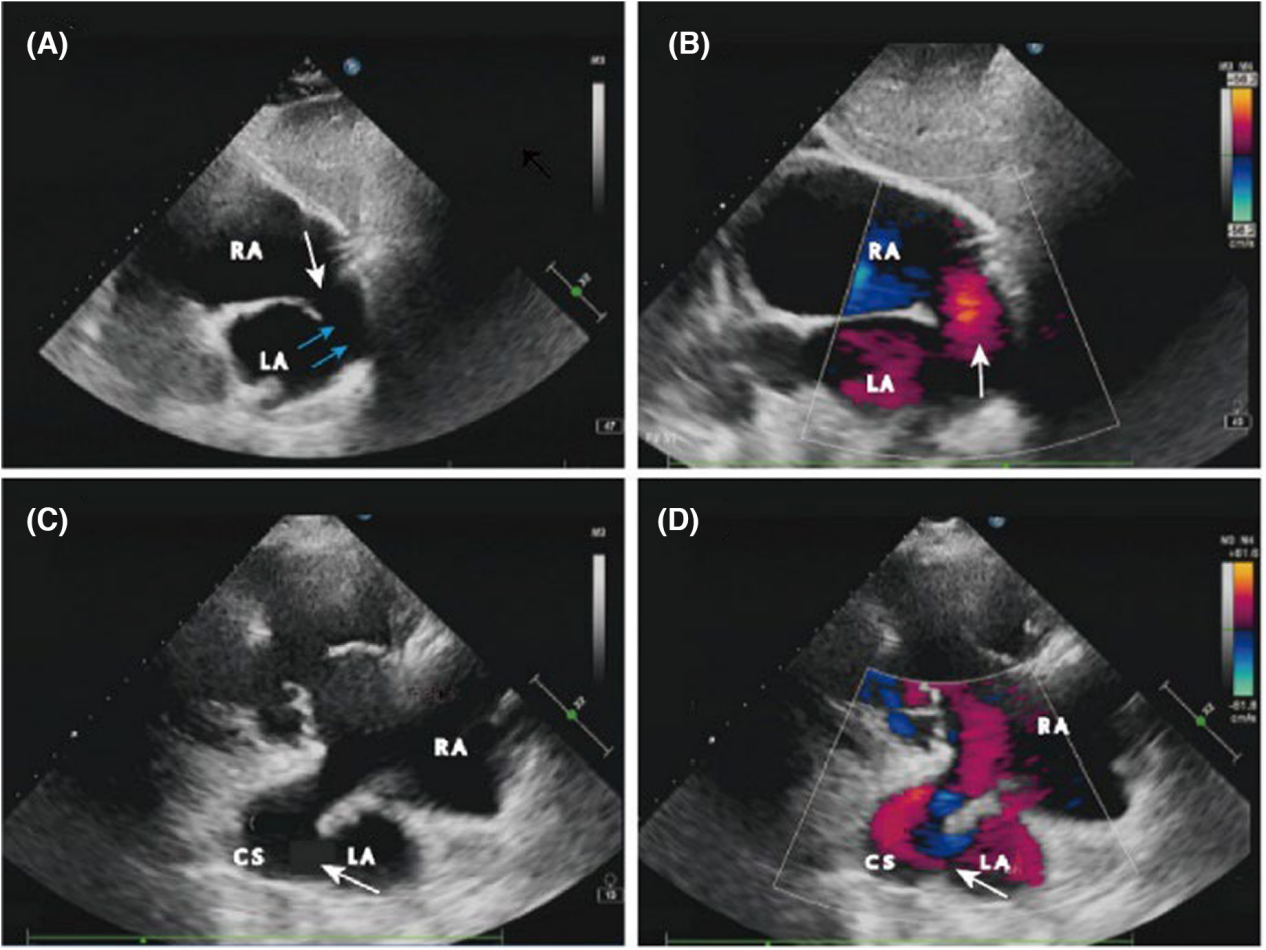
Preoperative two‐dimensional echocardiography. (A, B). Subxiphoid two‐atrial view: (A). The two‐dimensional echocardiogram shows the total absence of the sinus wall between the LA and CS and the interrupted echo of the posterior lower part of the atrial septum (blue arrows show the sinus wall, white arrows show the site of the septal defect); (B). Color Doppler shows the direct flow of blood from the LA into RA through the coronary venous sinus defect and the atrial septal defect (white arrows show); (C, D). Section of the right ventricular inflow view: (C). Two‐dimensional ultrasound shows dilated coronary sinus and coronary sinus wall defect (shown by white arrows); (D) color Doppler shows blood flow from the LA directly into RA through the CS sinus wall defect (shown by white arrows). (LA, left atrium; RA, right atrium; CS, coronary sinus).
